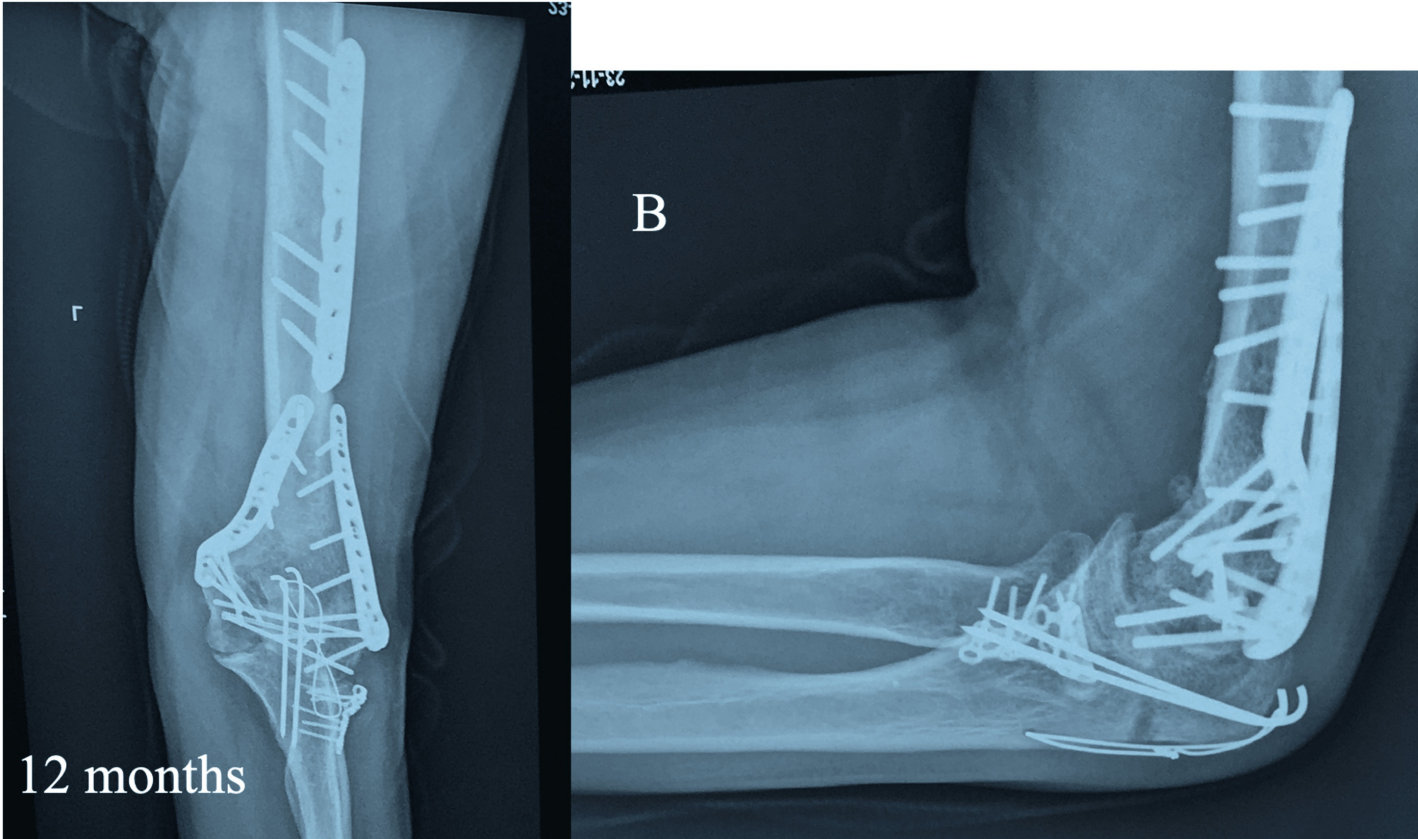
Radiographs at 12 months with the humerus in anteroposterior (left radiograph) and lateral positions in order to depict the union of all fractures.
